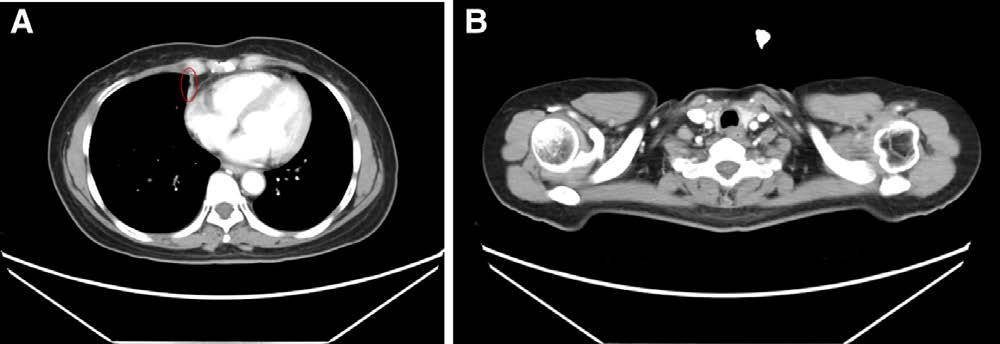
Chest CT follow-up on July 8, 2022. (A) Continued reduction in the right lung lesion; (B) no detectable metastatic lesion in the right supraclavicular lymph node. CT = computed tomography.
